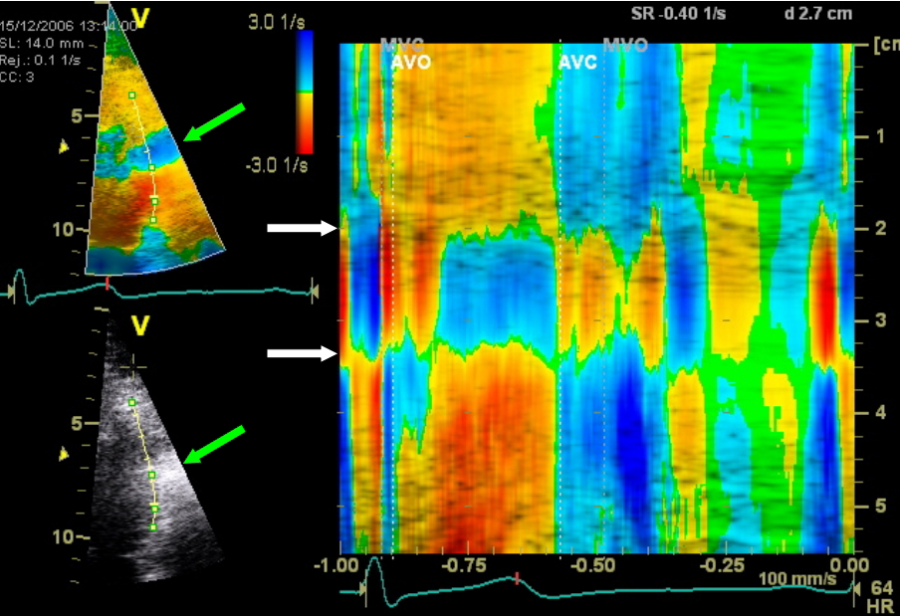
Impact of a stationary artifact on Doppler derived SR. TDI derived color coded M-mode of strain-rate in the lateral wall of one cardiac cycle. A stationary artifact (between white arrows) disturbs the velocity derived parameters. Note the reversal of the strain rate in the artifact and the overestimated SR below the artifact. This pattern closely resembles pathology, although the reversal in each phase (also during the atrial contraction) suggest an artifact which is also clearly seen on the B-mode images in this case (green arrows). Bottom = base; top = apex; yellow-red = negative SR; green = no SR; Blue = positive SR.
